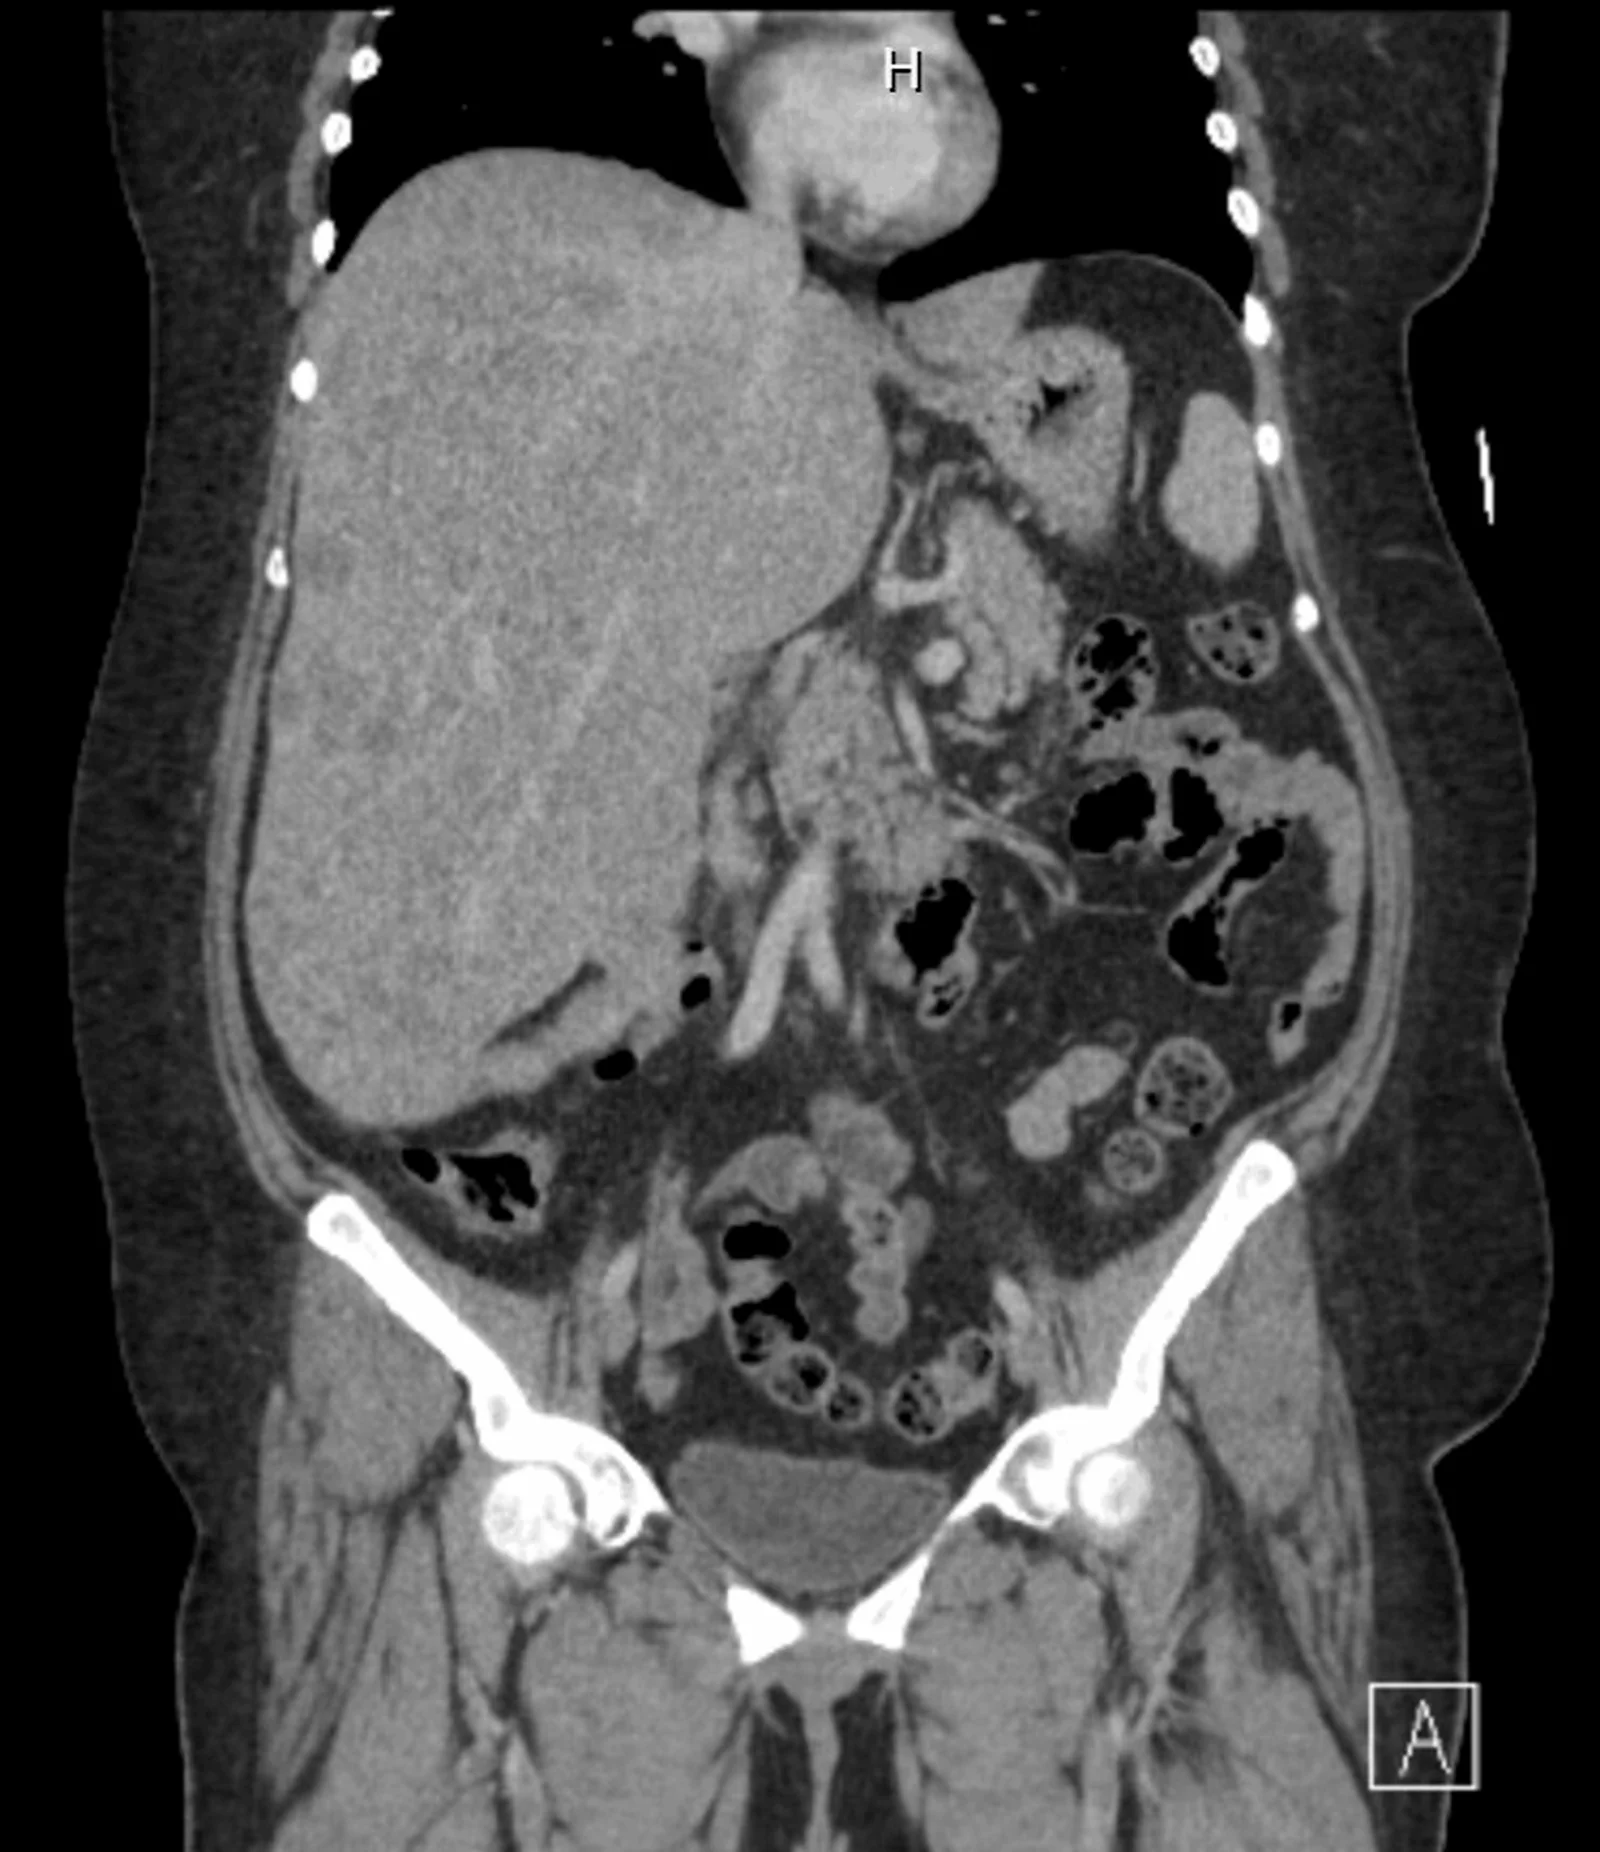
Coronal CT of the abdomen and pelvis demonstrating profound hepatomegaly with multiple mass lesions
